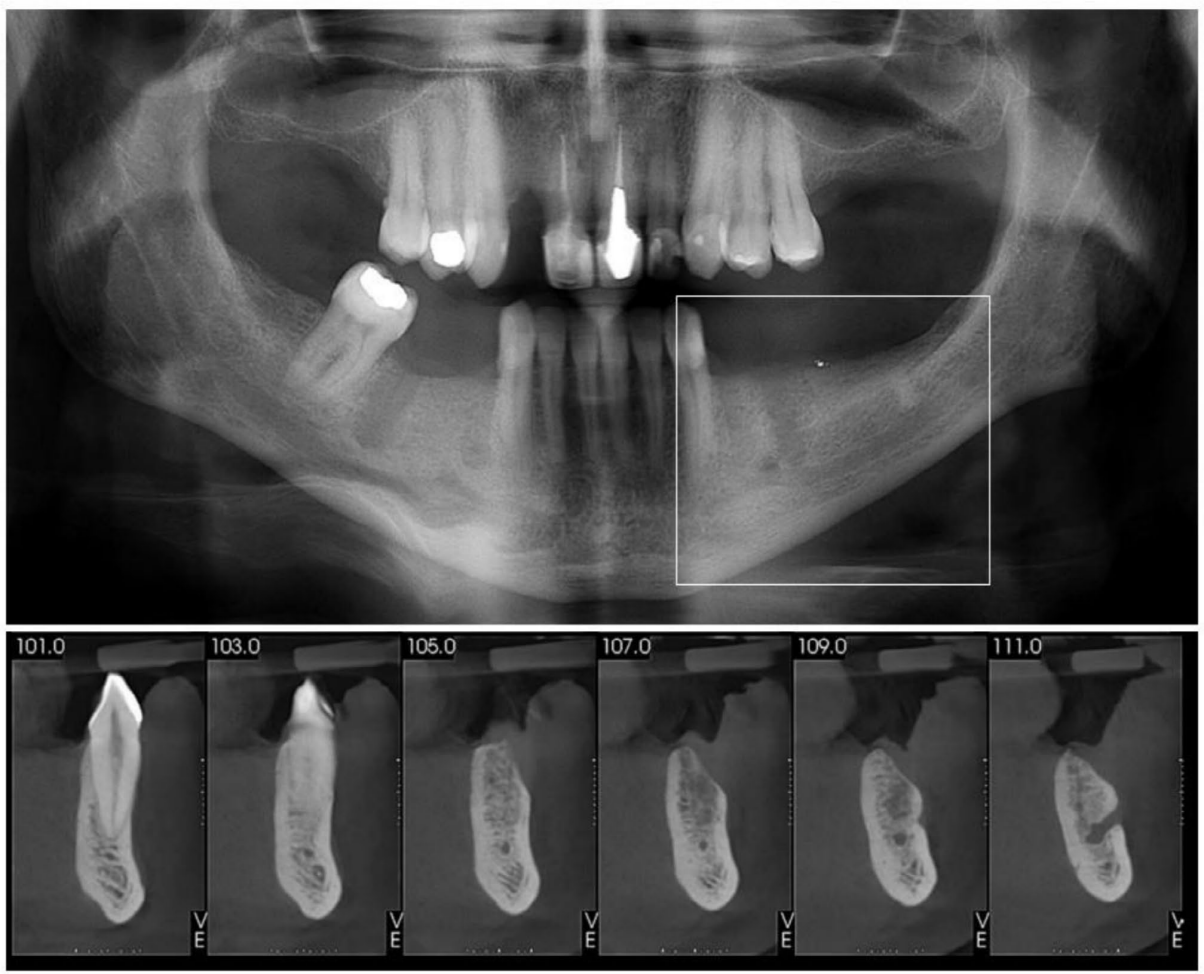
Initial radiographic and CBCT aspect.
